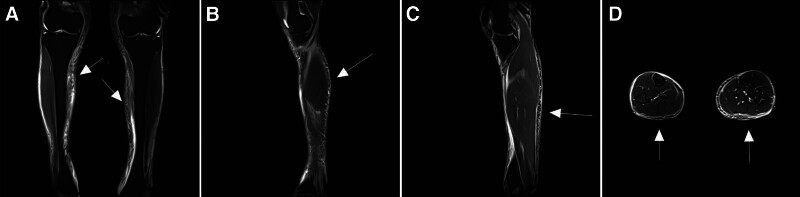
Lower limb MRI. (A) Coronal T2WI, both calves. (B) Sagittal T2WI, right calf. (C) Sagittal T2WI, left calf. (D) Axial T2WI, both calves. MRI = magnetic resonance imaging, T2WI = T2-weighted imaging.
